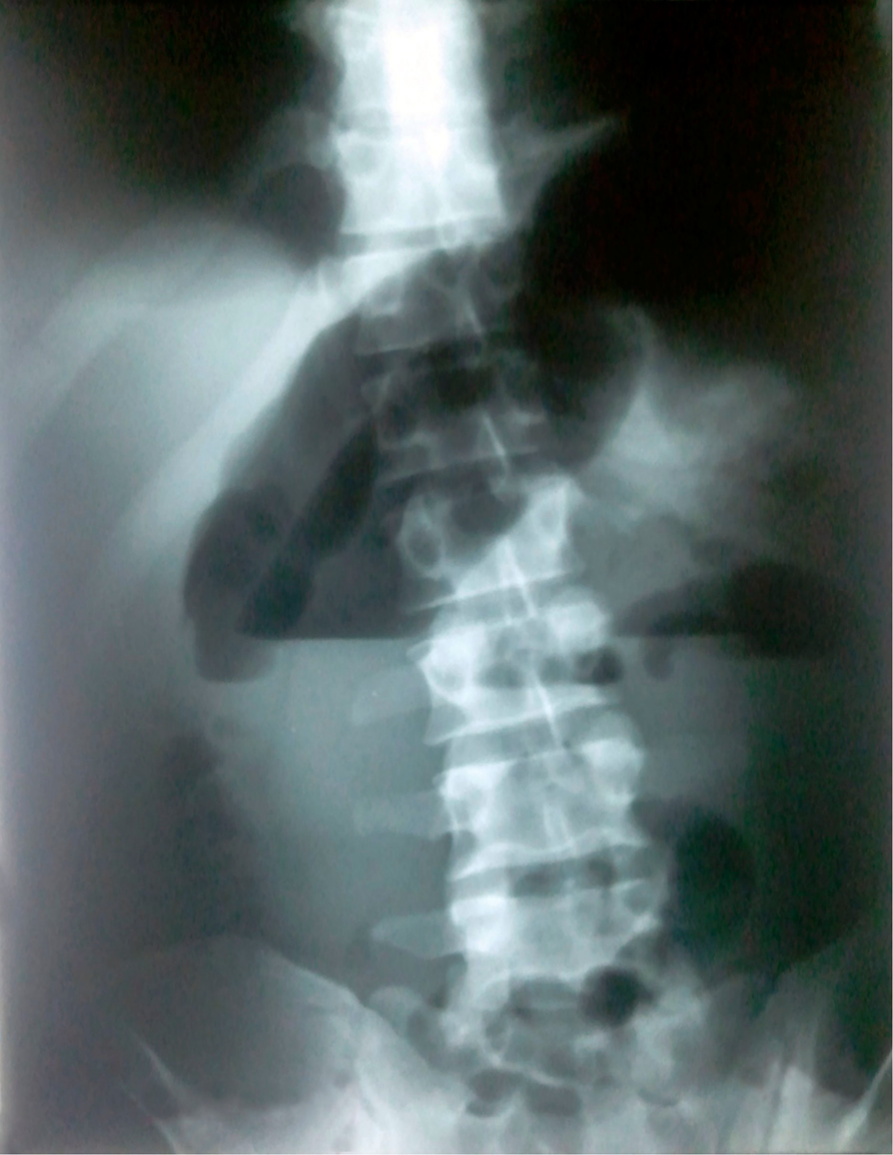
Abdominal X-ray revealing several air-fluid levels of the small bowel and a large air-fluid level of the sigmoid colon.
